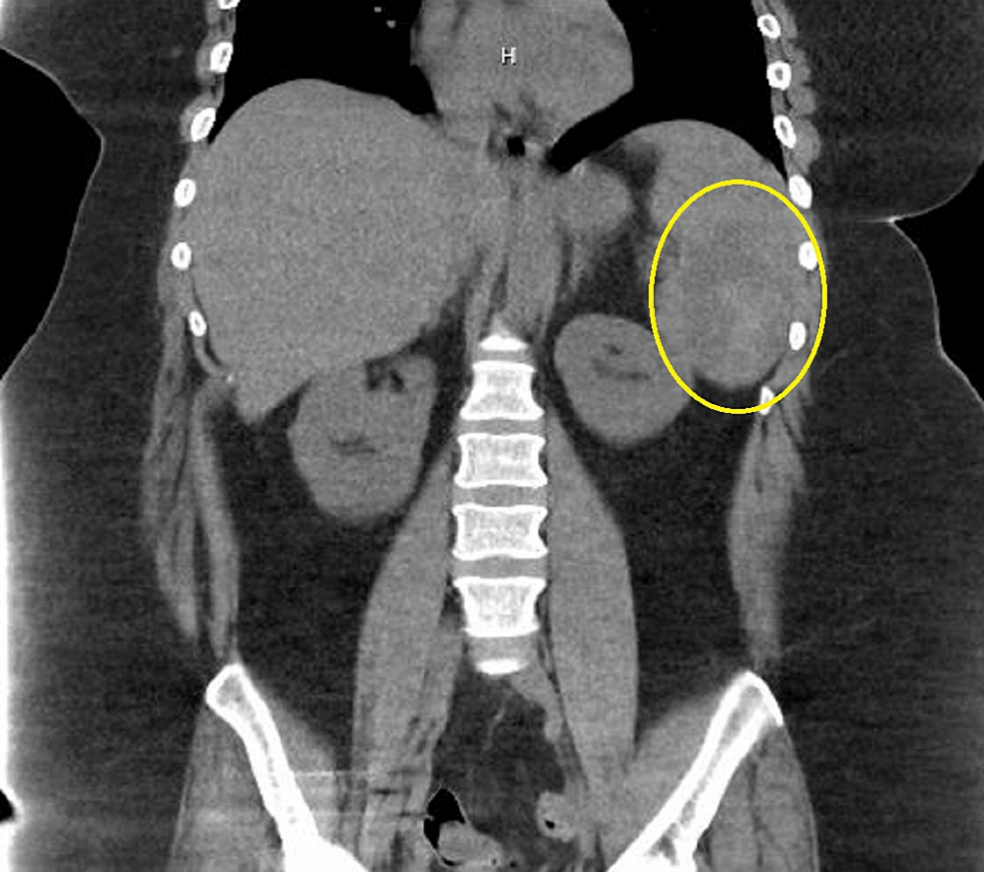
Coronal section of CT of the abdomen and pelvis showing an ill-defined lesion in the spleen (yellow circle) CT, computed tomography
